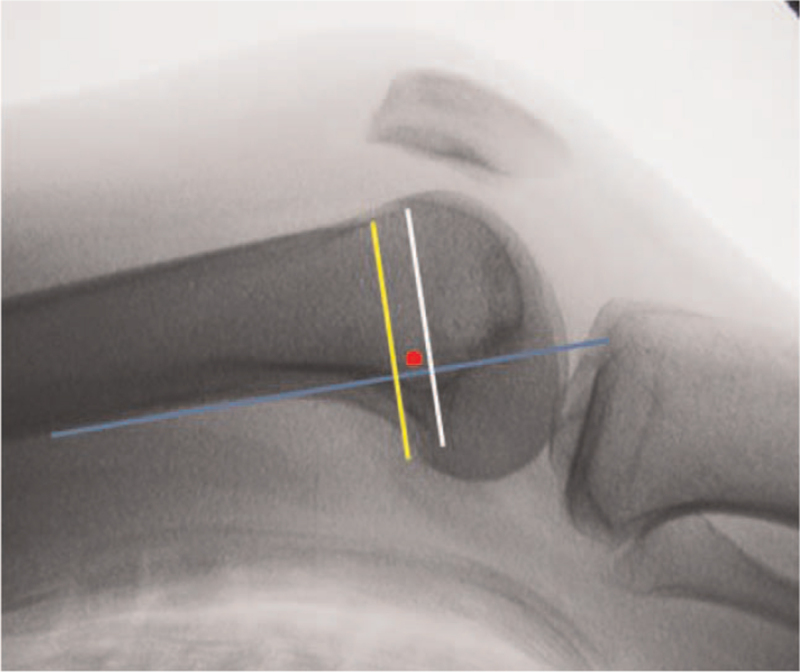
The specific location of the anatomic femoral attachment point on the standard lateral radiograph.
